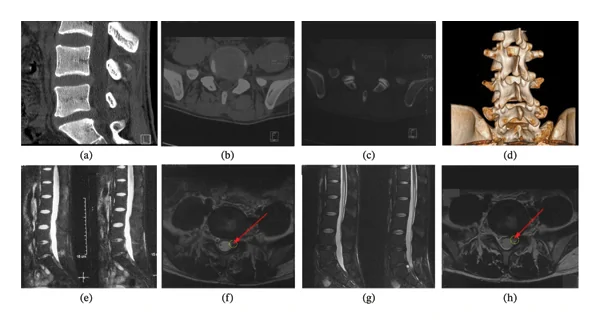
Postoperative follow‐up: (a–d) lumbar spine CT captured at 1 week postoperatively, consisting of (a) sagittal view, (b) axial soft‐tissue window, (c) axial bone window, and (d) 3D reconstruction showing optimal surgical entry and intact facet joint stability. (e, f) Lumbar spine MRI at 1 week postoperatively, displaying (e) sagittal and (f) axial views. (g, h) Lumbar spine MRI at 1 month postoperatively, displaying (g) sagittal and (h) axial views. Notably, on both the 1‐week (f) and 1‐month (h) postoperative axial sequences, the yellow circles and red arrows delineate the thoroughly decompressed nerve roots and the completely restored patency of the lateral recess.
